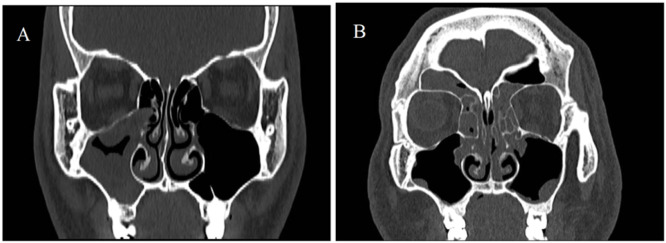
(A) CRSsNP CT imaging features. (B) RSwNP CT imaging features.
